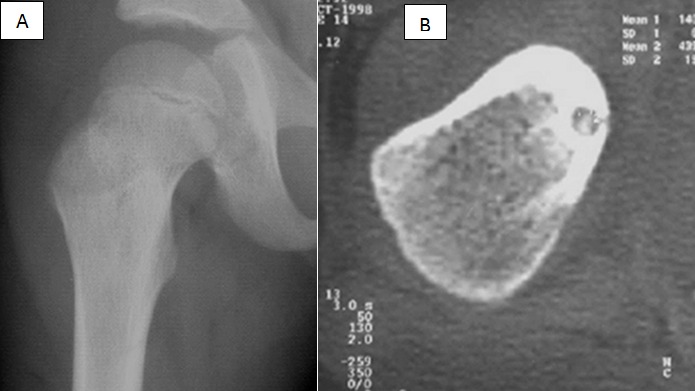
Ostéome ostéoïde, radiographie (A), ostéocondensation du col fémoral TDM (B): image typique du nidus
